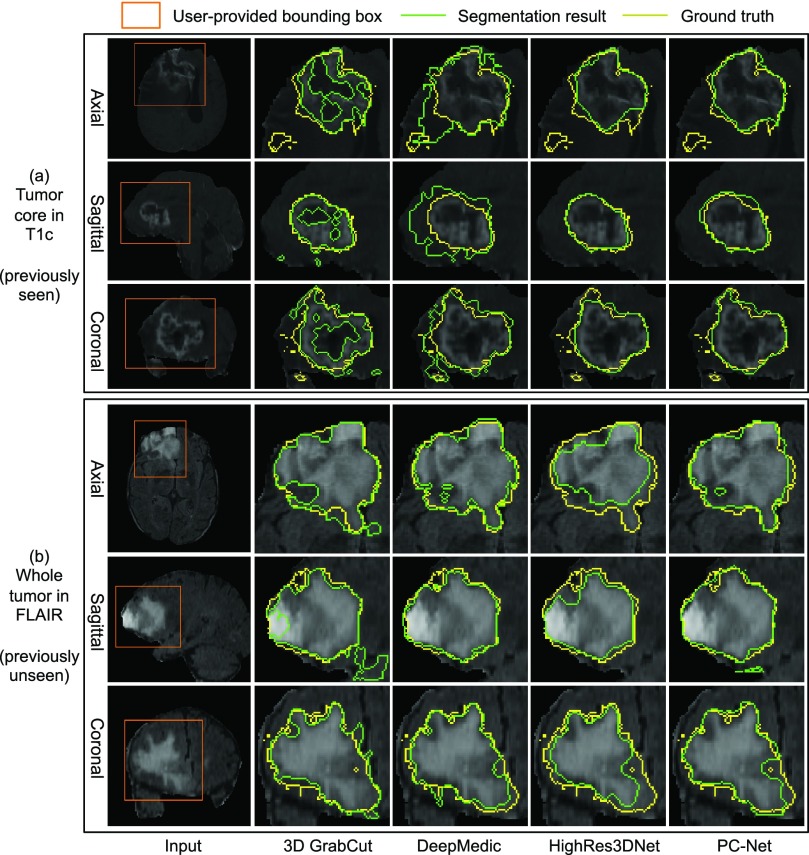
Visual comparison of initial segmentation of brain tumors from a 3D bounding box. The whole tumor in FLAIR is previously unseen in the training set. All these methods use the same bounding box for each test image.
